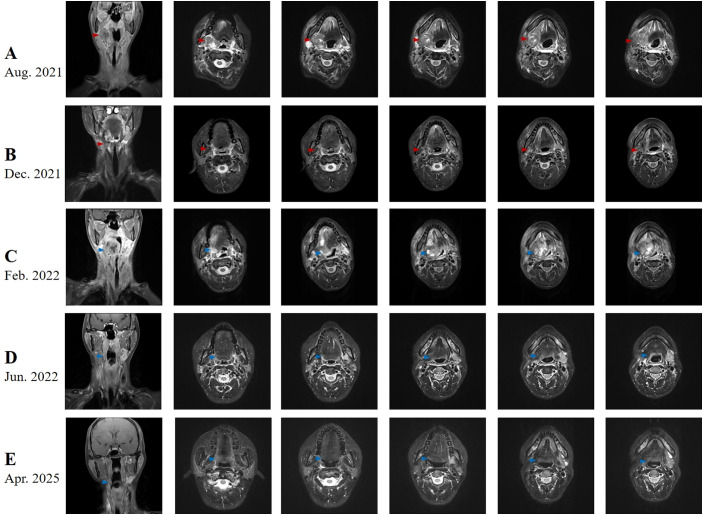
Radiographic imaging of advanced unknown primary carcinoma of the head and neck (UPCHN) during therapy and follow-up. (A) August 2021: Initial scan showing a right submandibular mass (red arrows). (B) December 2021: The disappearance of all target lesions (red arrows) following neck dissection and chemoradiotherapy. (C) February 2022: A newly developed irregular mass in the right oropharyngeal region (blue arrows). (D) June 2022: The disappearance of all target lesions (red arrows) after treatment with the weekly PCC regimen (blue arrows). (E) April 2025: Most recent MRI showing no signs of tumor recurrence or progression (blue arrows).
